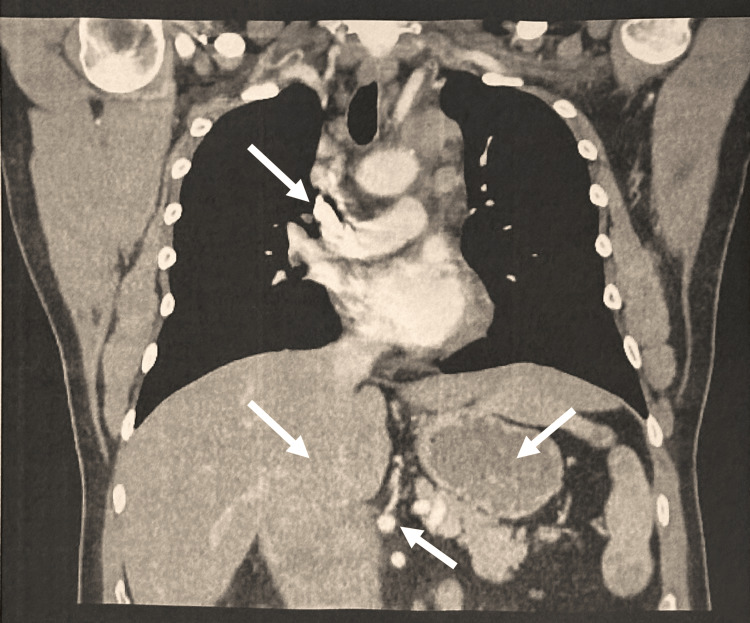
CT Chest (Month 2) Extensive mediastinal and supraclavicular nodal metastases.
